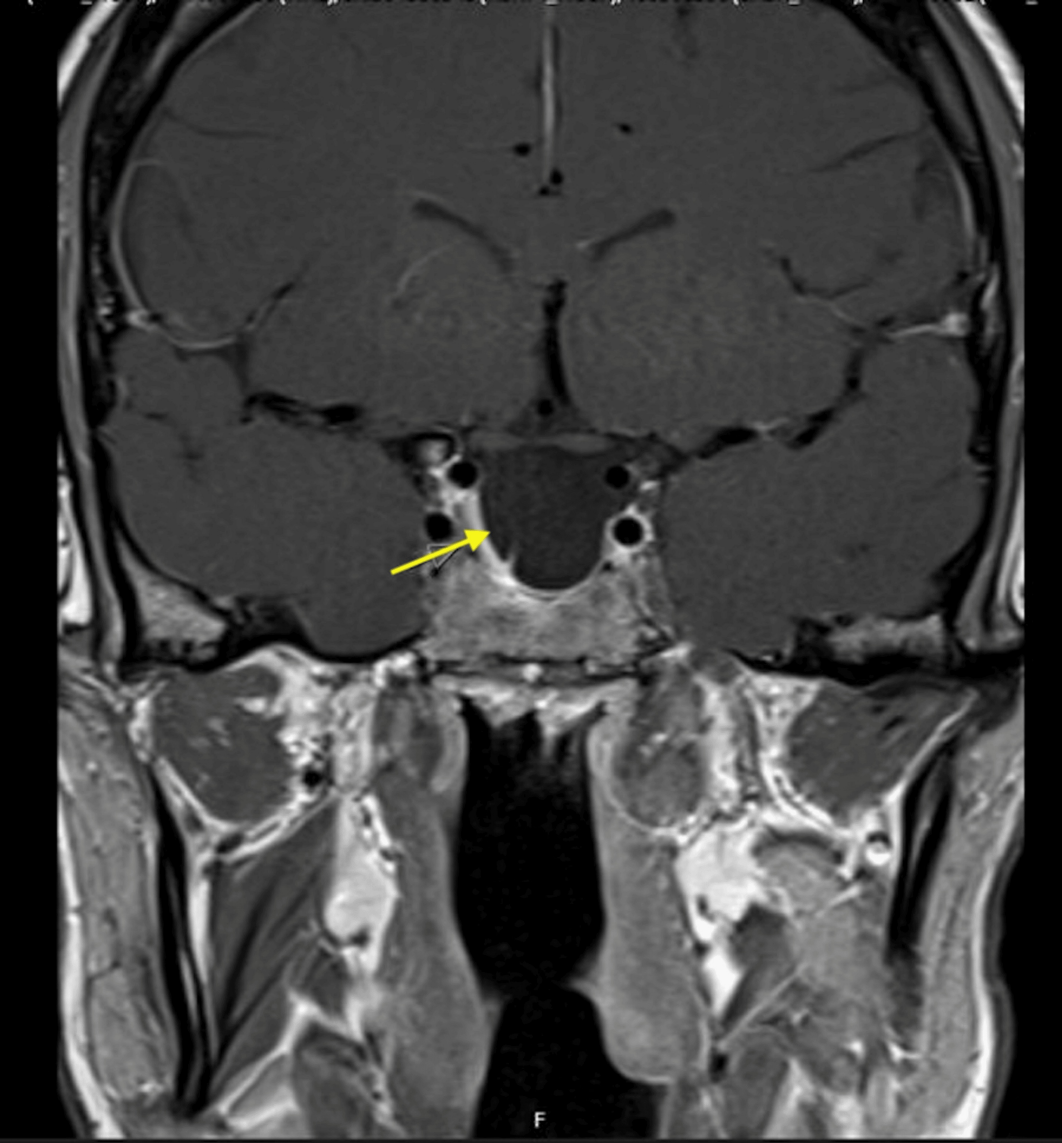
Coronal view of the postoperative pituitary MRI Arrow pointing towards a partial empty sella in a post-operative pituitary MRI following complete excision of a pituitary adenoma.
